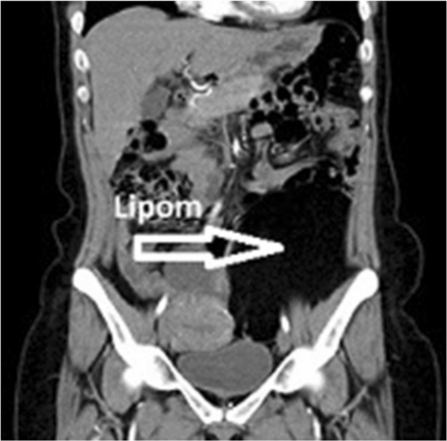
CT image of lipoma in the lower left quadrant.
